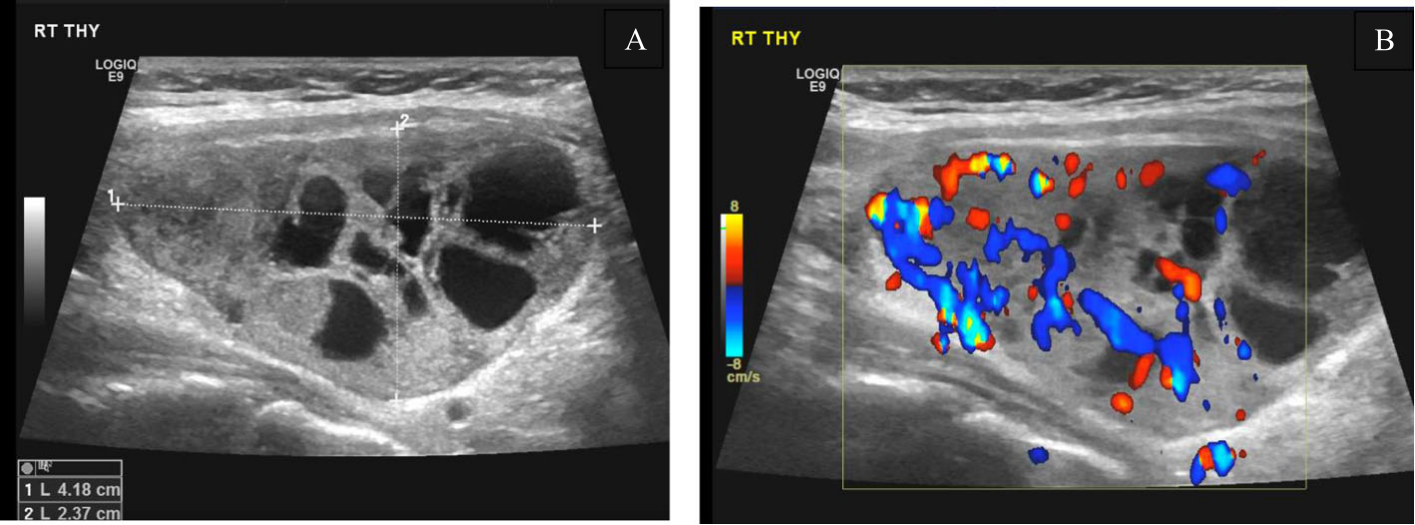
Ultrasound of the neck showing complex nodule of the right lobe with solid and cystic components (a) and vascularity (b)
